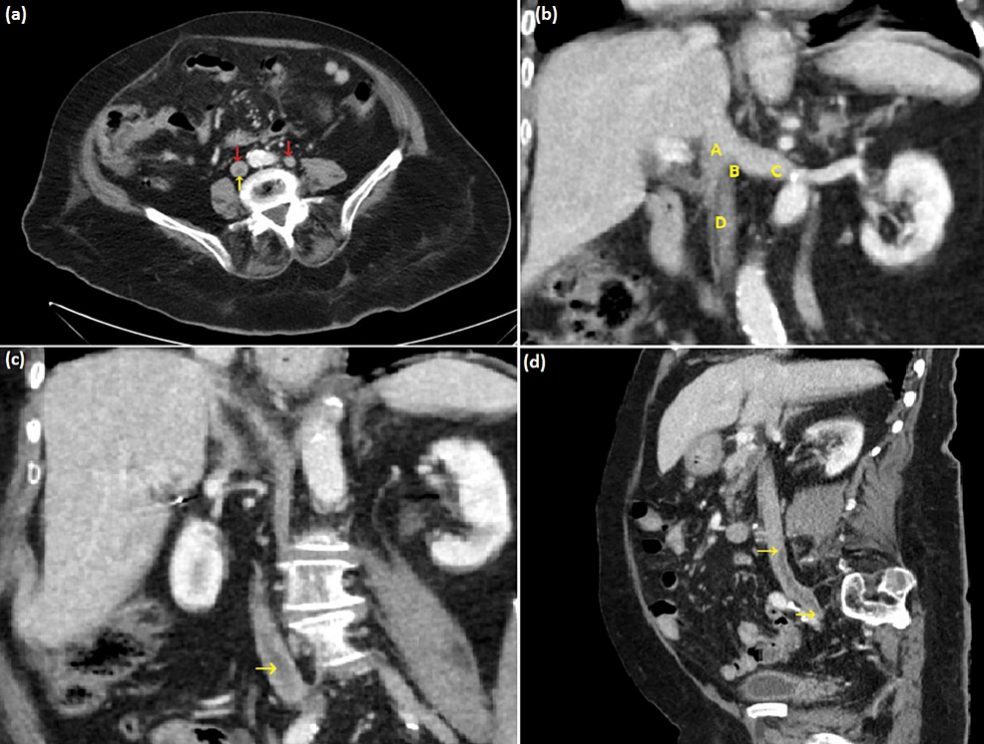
Duplication of the IVC (a) Axial CT image demonstrating a duplicated IVC (red arrows) with thrombus noted in the right IVC (yellow arrow); (b) Coronal view depicting (A) common IVC, (B) IVC confluence where both right and left IVC meet, (C) left IVC, and (D) right IVC; (c) Coronal view showing a central filling defect (yellow arrow) within the infrarenal portion of the right IVC; (d) Sagittal view demonstrating the extent of the thrombus (yellow arrows). It extends from the confluence of the right external and internal iliac veins to the infrarenal right IVC at the level of the L3 vertebral lower endplate CT: computed tomography; IVC: inferior vena cava
